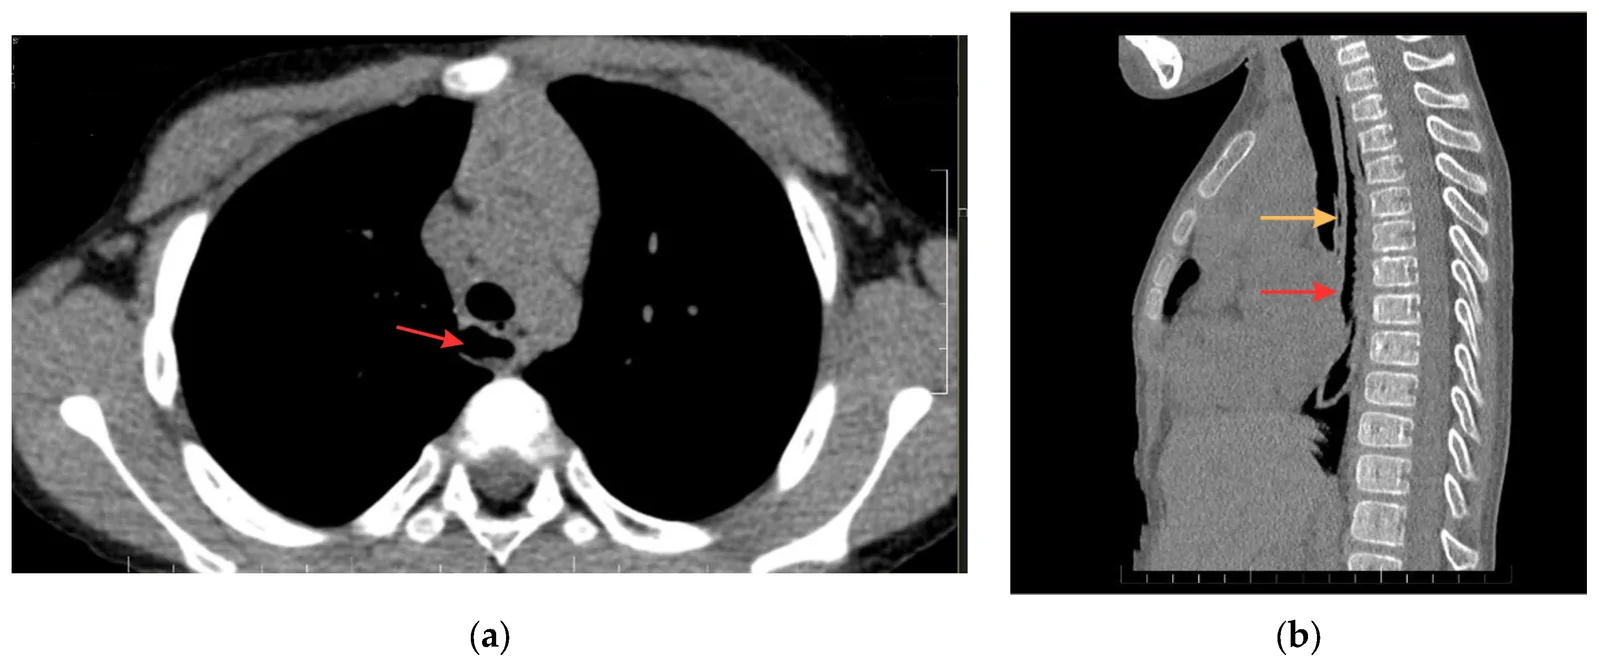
Thoracic CT scan in a 6-year-old boy showing esophageal dilatation (red arrows) and a posterior tracheal air-filled tract (yellow arrow) suspicious for recurrent or residual tracheoesophageal fistula. (a) Transverse plane; (b) sagittal plane.
